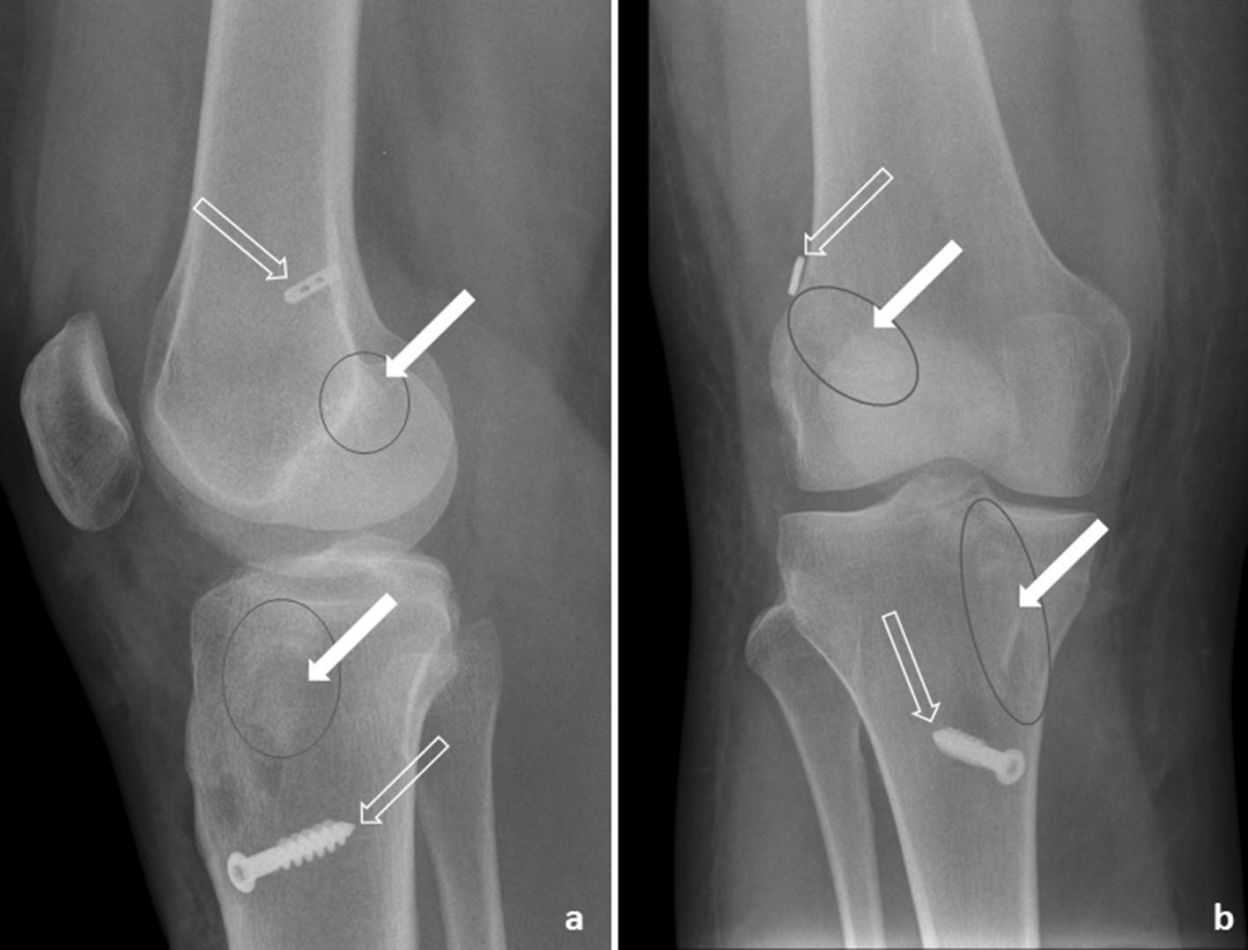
Xray 2 days after surgery (*Press-Fit-Hybrid®*-technique): a lateral view; b anterior/ posterior view; clear arrow: button and screw, white arrow bone block, for better visibility bone blocks are marked with black circles
